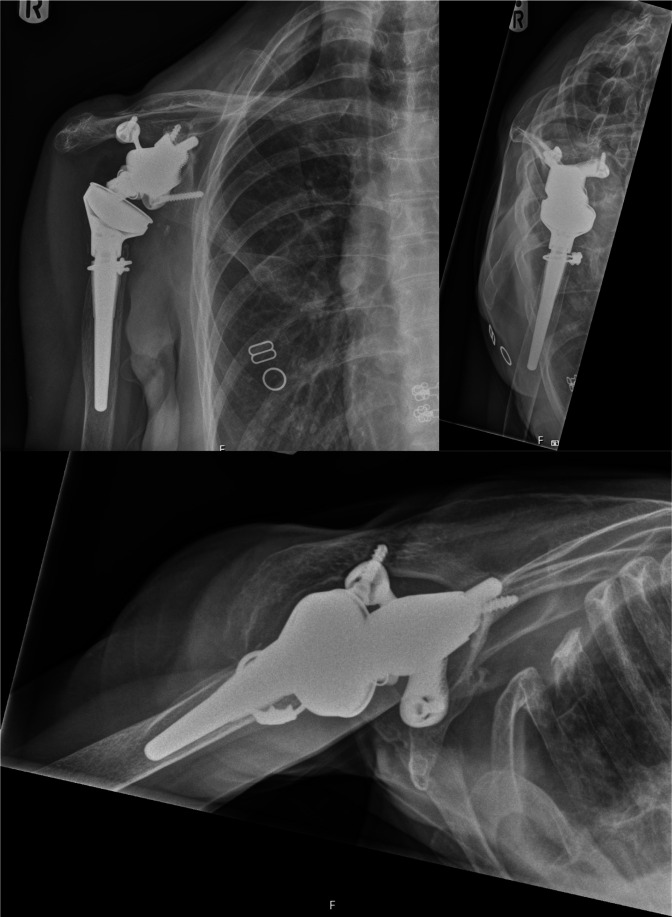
X-ray images taken 12 months post-operatively, demonstrating satisfactory prosthesis position.
